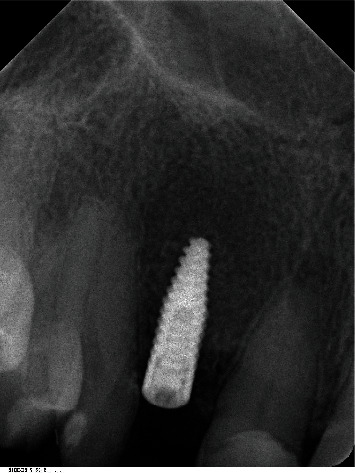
Dental X-ray after dental implantation.
